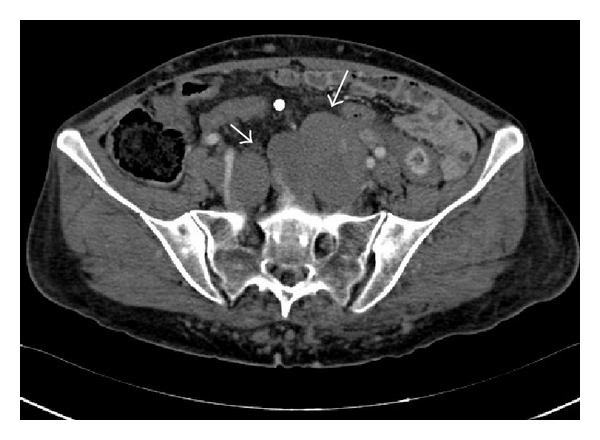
Axial contrast enhanced (arterial phase) CT of the pelvis demonstrating abnormal dilated lymphatic channels (arrows).
